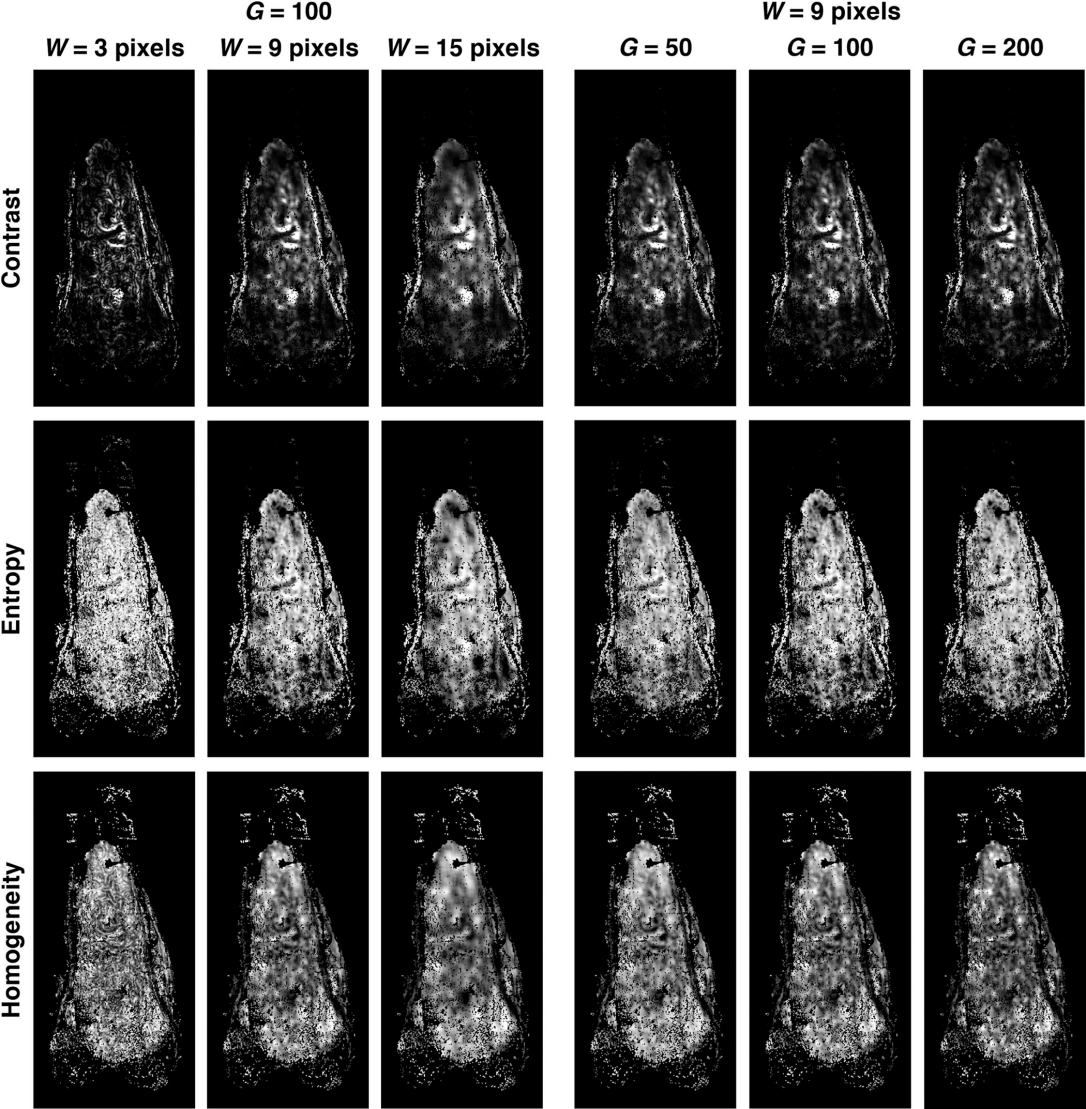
Effects of window size *W* and number of gray levels *G* on Haralick texture features. Images show the differences in texture features, such as contrast, entropy, and homogeneity, computed for the tumor AOI_hist_ in the post-contrast T1-weighted MR image as window size *W* varies (from 3 to 9 to 15 pixels) while keeping the number of gray levels constant at *G* = 100. No visible differences can be observed in the texture features when the number of gray levels *G* changes from 50 to 100 to 200 while the window size remains constant at *W* = 9 pixels.
